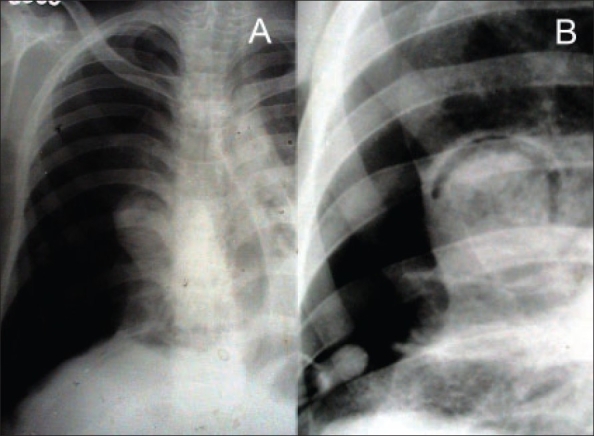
Chest roentgenogram PA view showing the right pneumothorax and the mycetoma with air-crescent sign; the tip of intercostal drain is also visible near the base.
